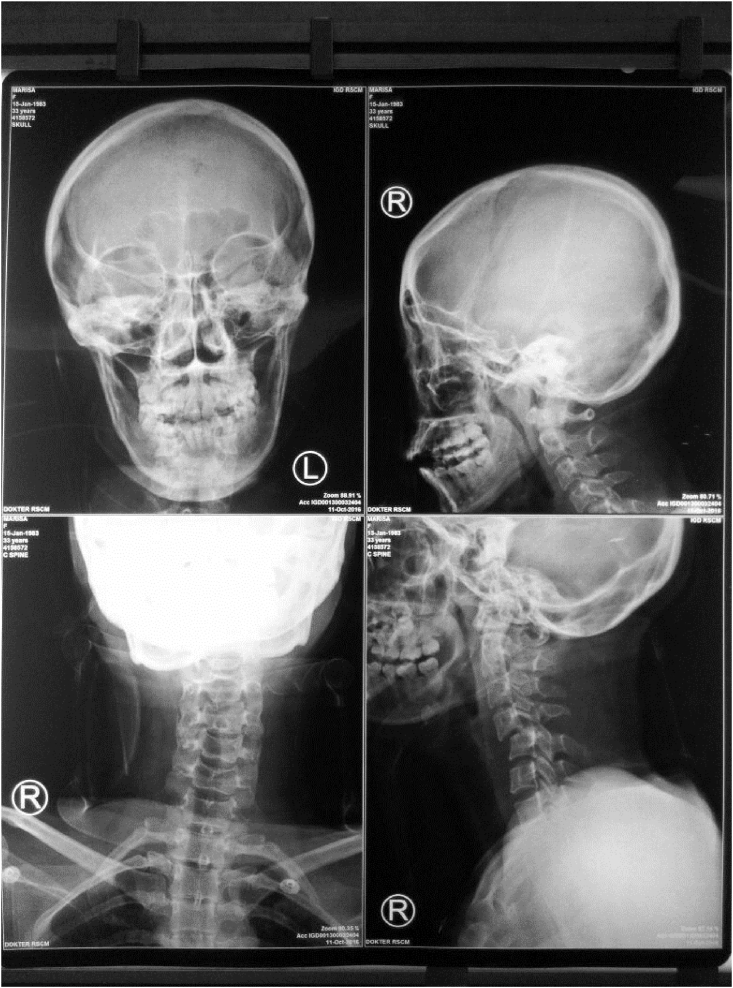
AP and Lateral radiograph of cervical spine.
